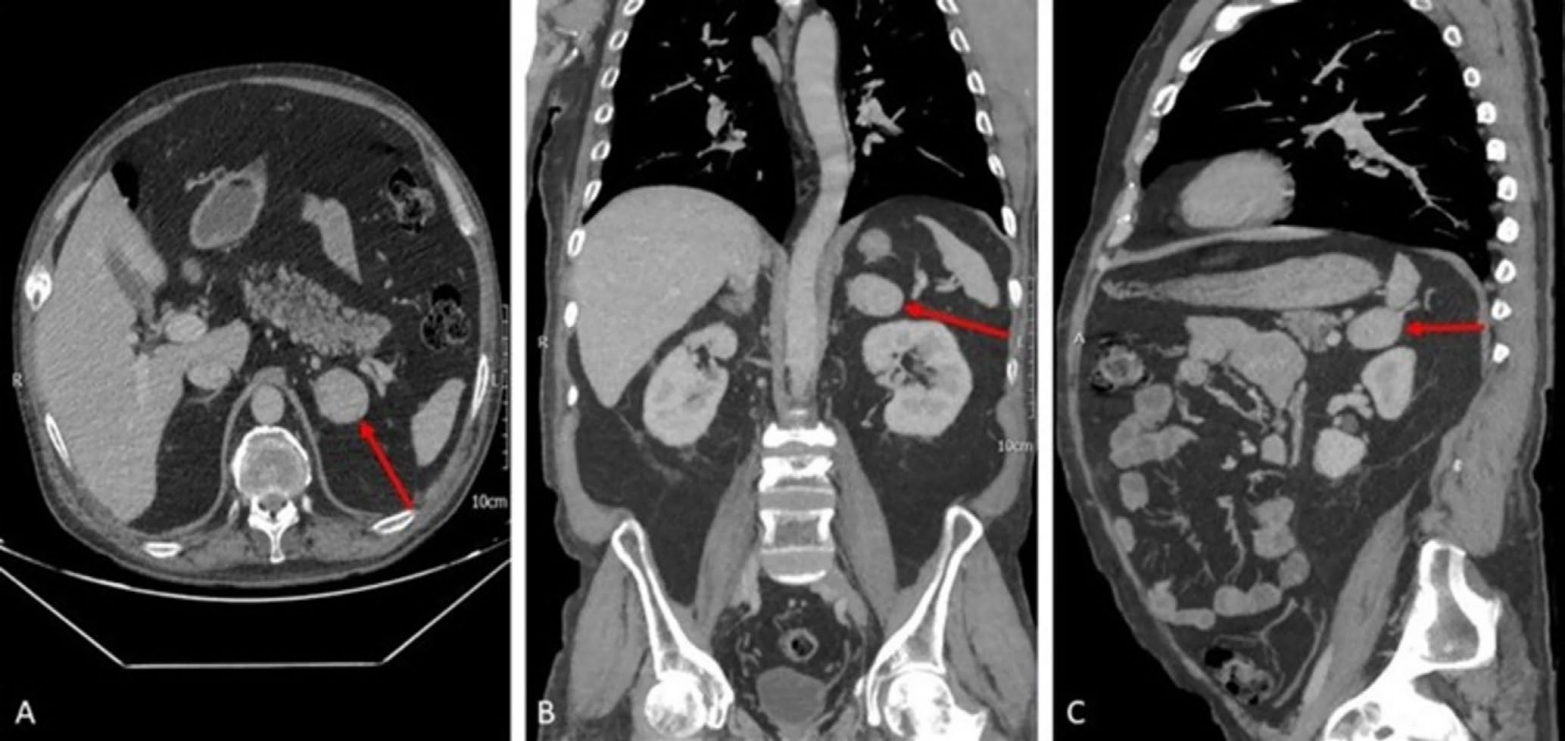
Abdominopelvic multidetector computed tomography (MDCT) with adrenal protocol showing a well‐defined lesion with an approximate size of 32.8 × 38.6 mm in the left adrenal gland; radiodensity 90 HU. (A) Transverse plane. (B) Coronal plane. (C) Sagittal plane.
